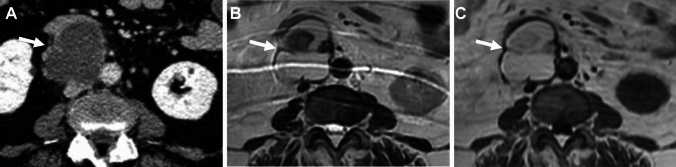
Retroperitoneal endometriosis (lymph node involvement). A 72-year-old with suspicion of abdominal mass on ultrasonography examination. A Contrast-enhanced CT reveals a cystic mass (arrow) located in the retroperitoneal space. The mass shows high signal intensity on both B T2-weighted image and C T1-weighted image suggesting its hemorrhagic contents with clots
